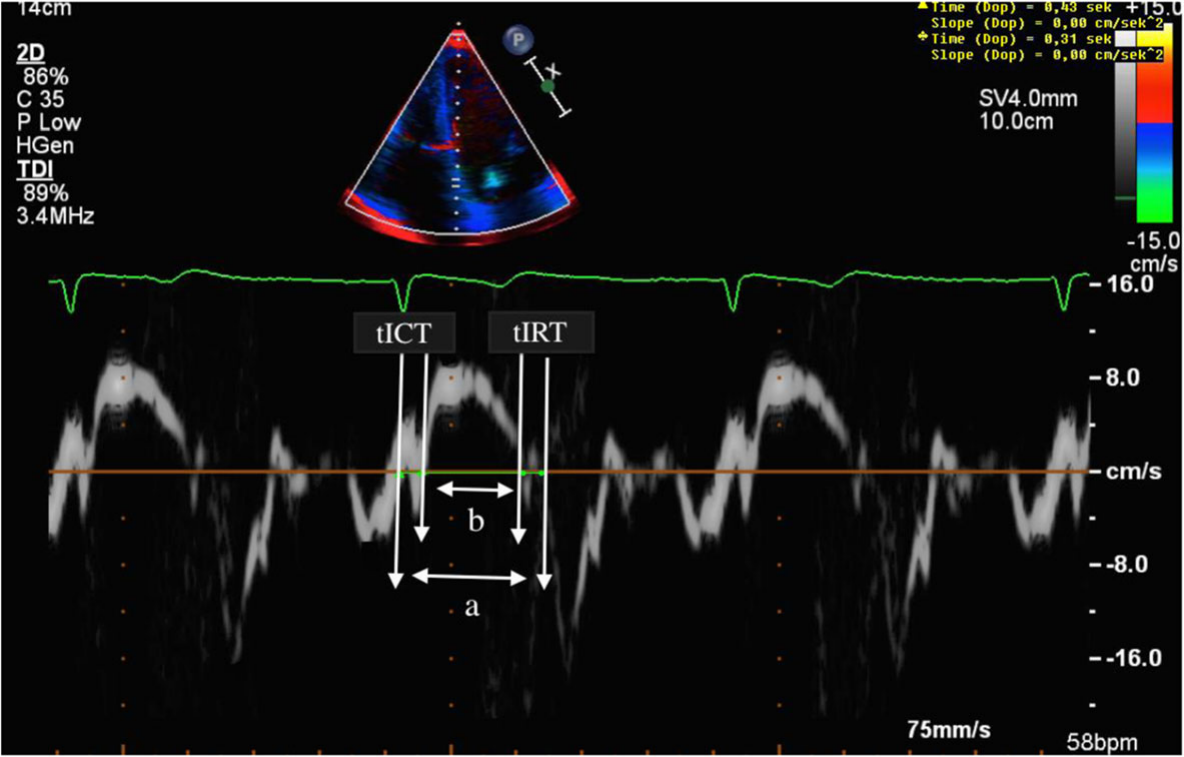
Myocardial performance index measured by tissue Doppler imaging. Time intervals by tissue Doppler imaging derived from septal annulus. tICT, tissue isovolumic contraction time; tIRT, tissue isovolumic relaxation time; a = isovolumic contraction time + isovolumic relaxation time + ejection time; b = ejection time. MPI = (a-b)/b
